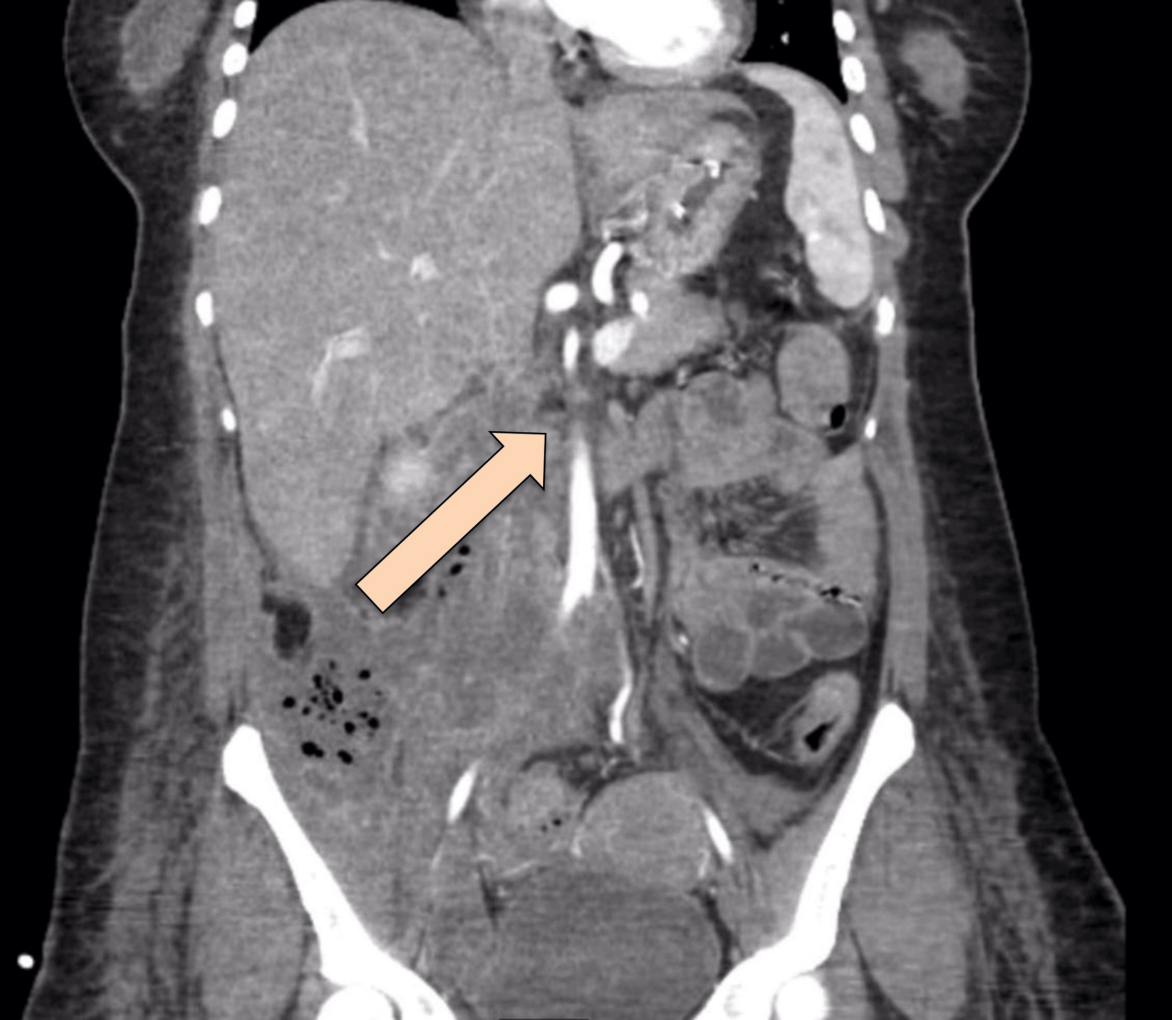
Follow-up coronal view of CT abdomen with IV contrast The arrow shows persistent communication between the para-duodenal collection and the posterior aspect of the distal CBD CT: computed tomography; IV: intravenous; CBD: common bile duct
